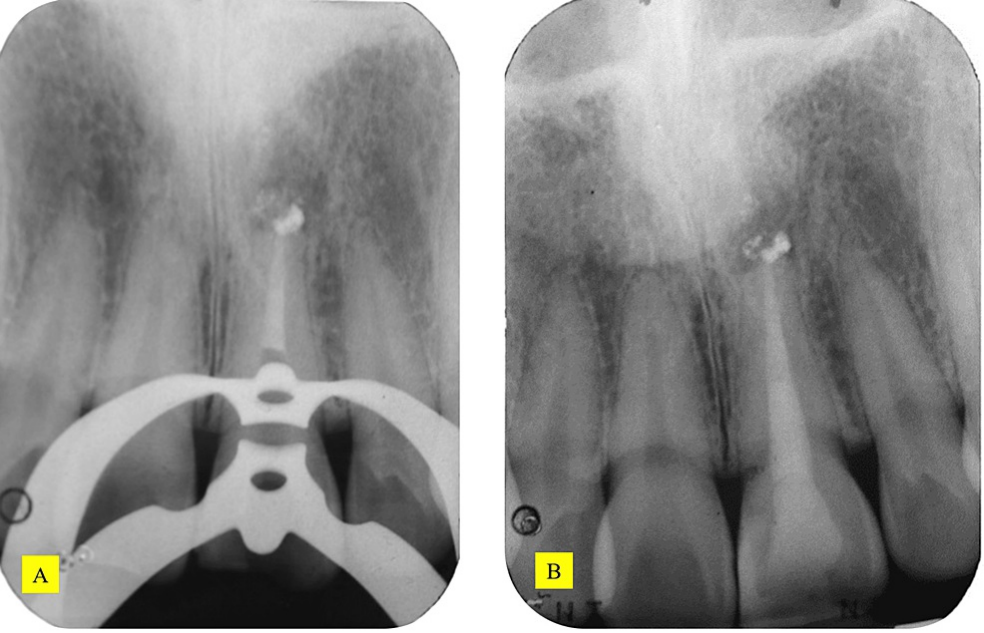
MTA placement radiograph and post-obturation radiograph of tooth 21 (A) MTA placement radiograph. (B) post-obturation radiograph
